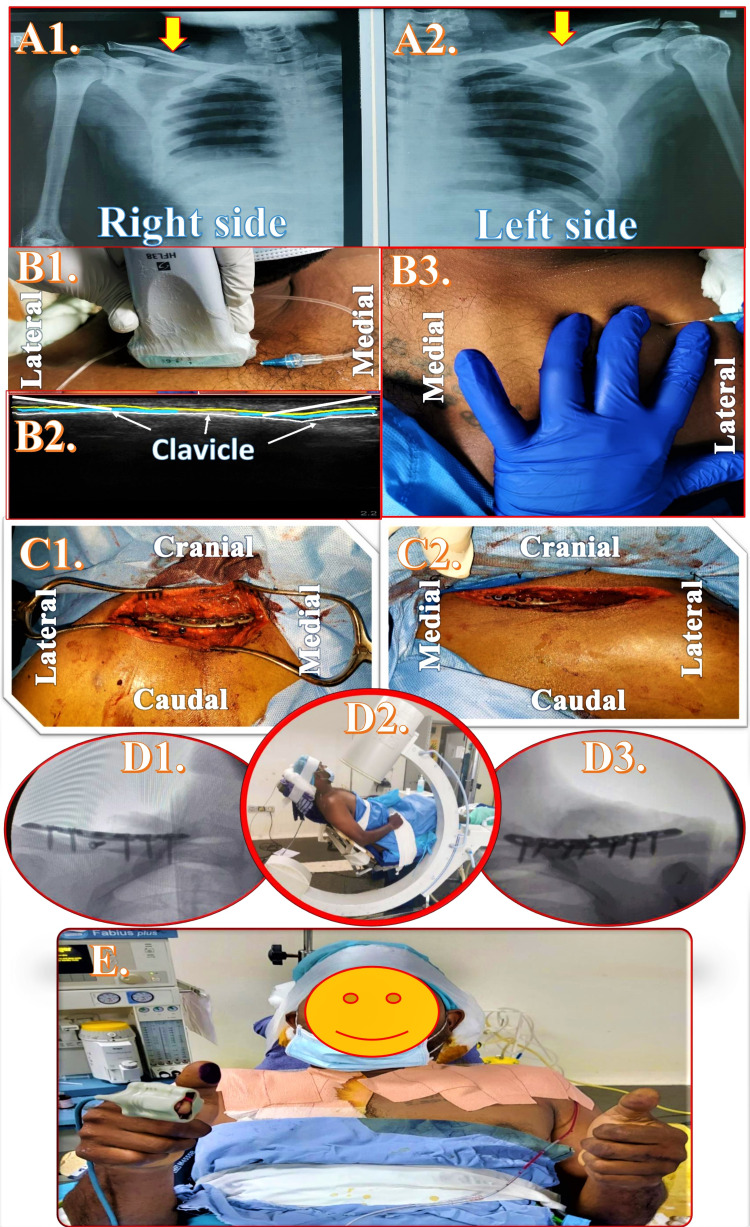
Radiographic and clinical images of the bilateral clavipectoral fascial plane block and bilateral clavicle fracture surgery A1, A2: Radiographic images of right and left clavicle fracture; B1, B2: Performance of ultrasound-guided clavipectoral fascial plane block; B3: Performance of landmark-guided clavipectoral fascial plane block; C1, C2: Surgical fields of bilateral clavicle surgery with implants in situ; D1, D2, D3: Patient positioning during surgery and intraoperative radiographic pictures; E: Smiling patient able to lift both upper extremities immediately after surgery
